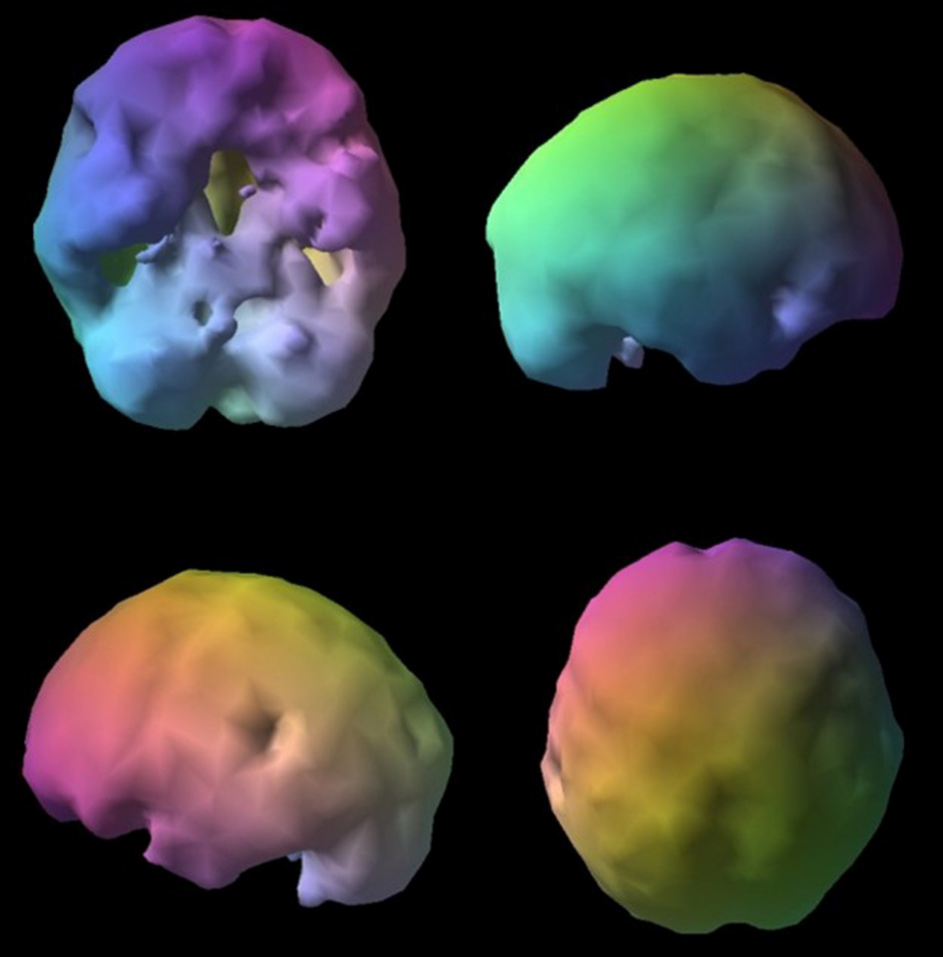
Surface SPECT images. Decreases in medial undersurface (see top left view).
